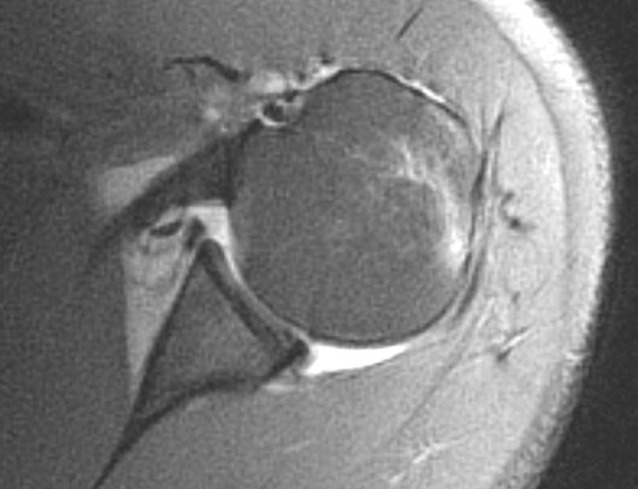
Axial T2-weighted magnetic resonance imaging sequence of a 36-year-old patient after a first-time shoulder dislocation. Given his age and the absence of any rotator cuff tear or other concomitant pathology, he was deemed low risk for re-dislocation. Therefore, nonoperative treatment was pursued, which was successful with no recurrent subluxation or dislocation
